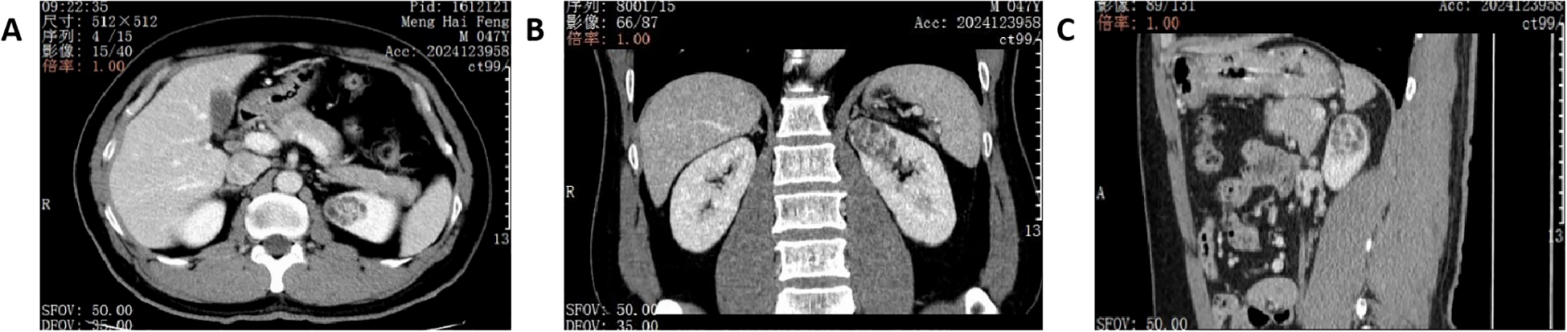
Renal CT images on admission. (A) axial plane of CT image. (B) coronal plane of CT image. (C) sagittal plane of CT image.
